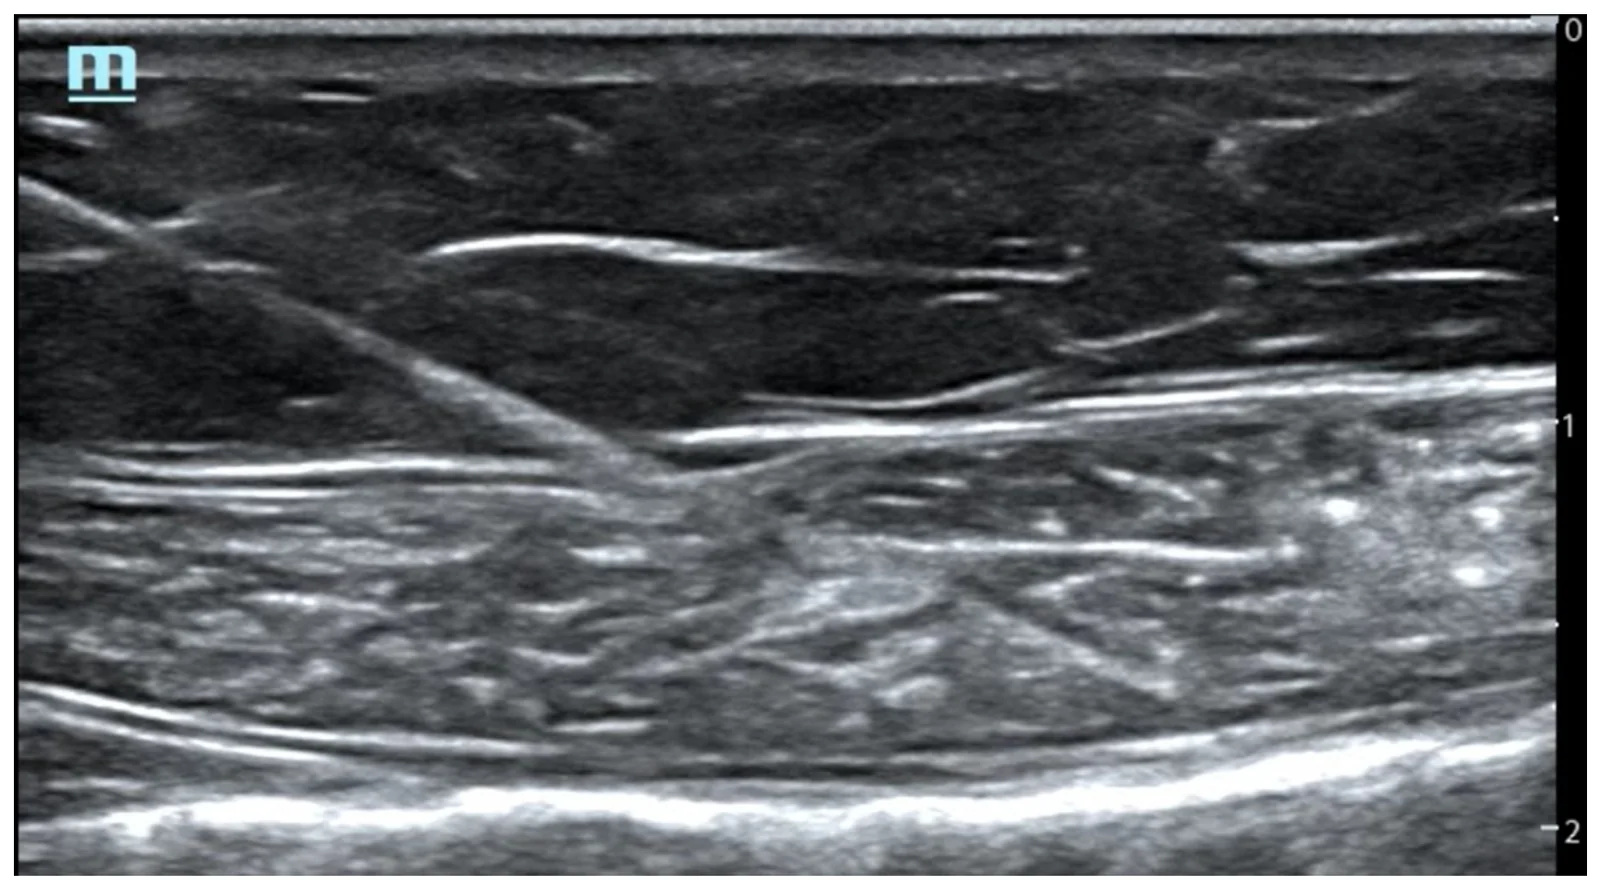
Ultrasound view of hydrodissection during RSB.
